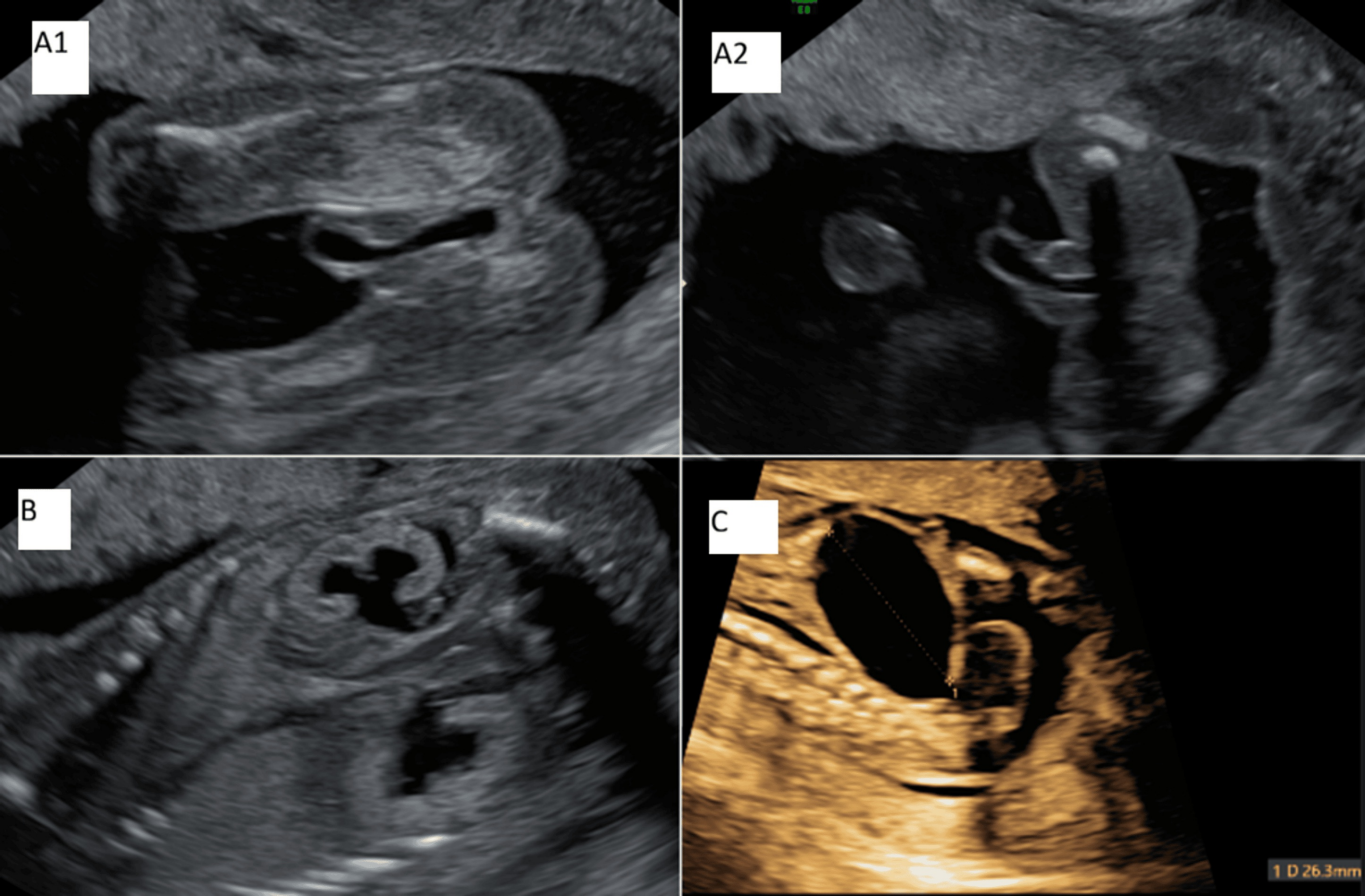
Ultrasound images of the fetus at 16 weeks and 6 days of gestation. (A1, A2) Penile urethra dilated to its distal end. (B) Bilateral hydronephrosis and hyperechoic kidneys. (C) Fighting bladder.
